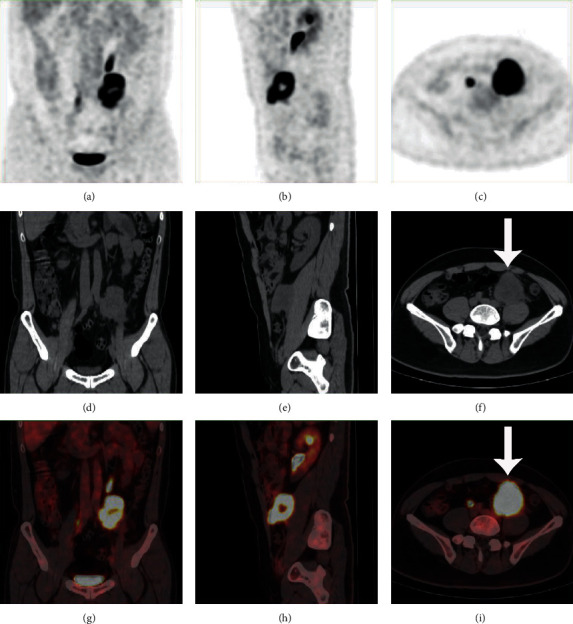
PET/CT images of a DDLPS patient with an SUVmax of 32.5 (white arrow).
